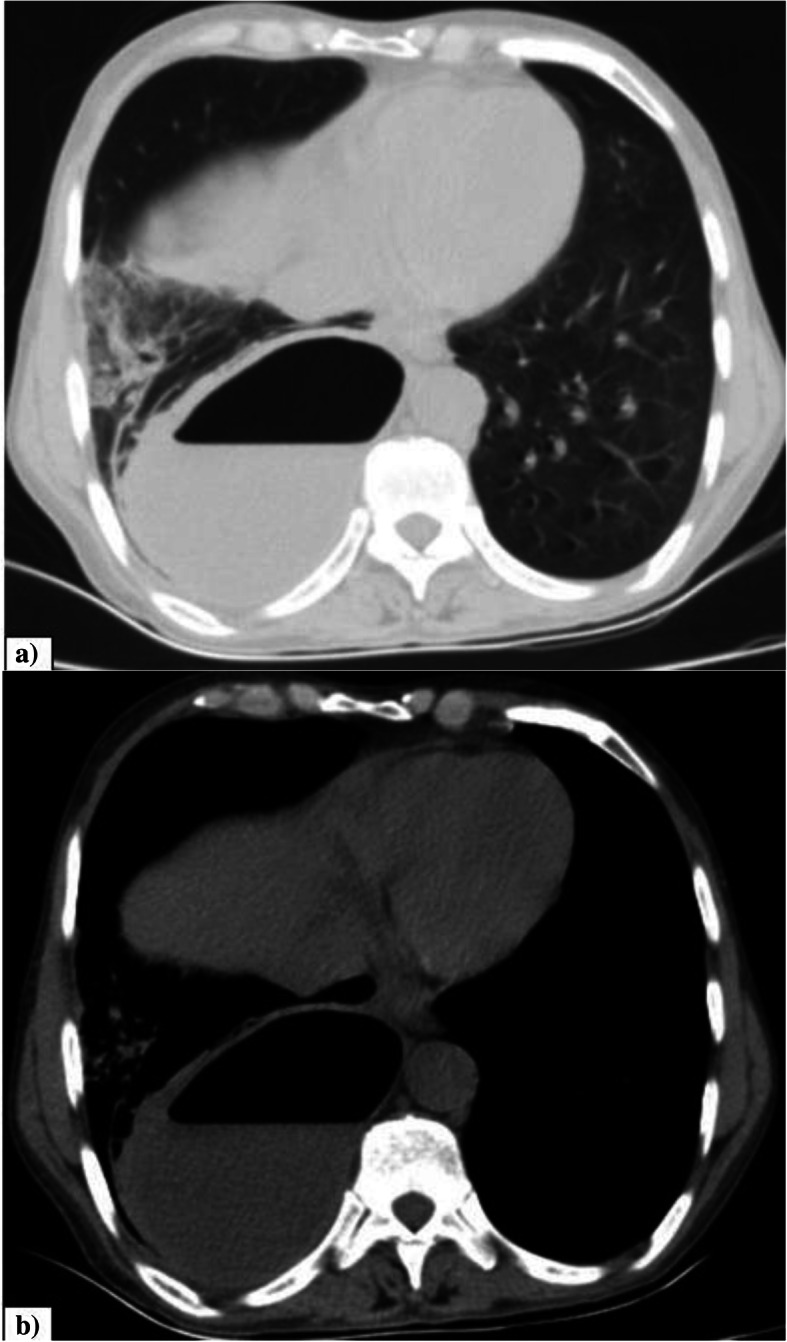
Empyema. a, b Axial CT thorax (lung window and mediastinal window) of a 63-year-old COVID pneumonia patient showing right sided loculated empyema. Adjacent areas of consolidation are also seen
